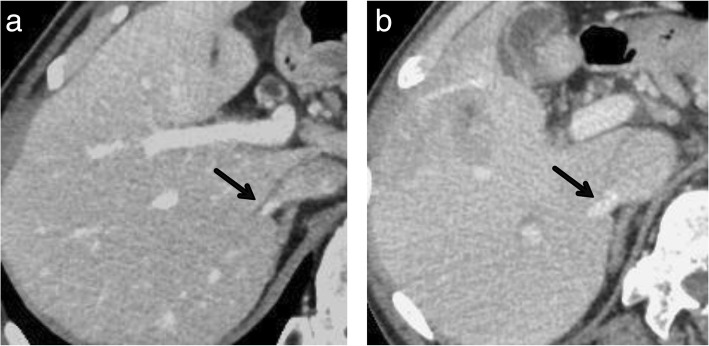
a Computed tomography obtained before embolization showed that the diameter of the inferior right hepatic vein (arrow) was 3.5 mm. b The diameter of the vein was enlarged to 7.7 mm 29 days after the right hepatic vein embolization
